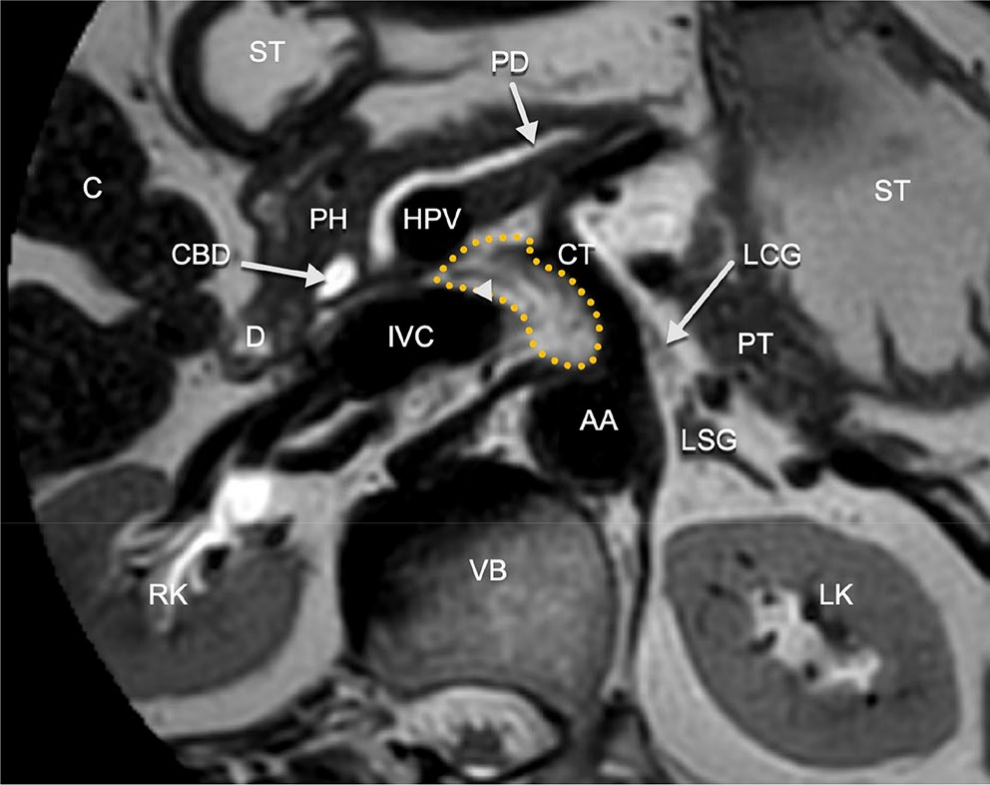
T2-weighted magnetic resonance image of the pancreatic head. The neural plexus contained by the retropancreatic fascia is visible (dotted yellow area in which several linear structures (arrow head) are visible corresponding to nerve fibers of the plexus). The left celiac ganglion (LCG) is visible to the left side of the celiac trunk (CT). AA: abdominal aorta, C: colon, CBD: common bile duct, D: duodenum (descending part), HPV: hepatic portal vein, IVC: inferior vena cava, LK: left kidney, RK: right kidney, LSG: left suprarenal gland, PD: pancreatic duct, PH: pancreatic head, PT: pancreatic tail, ST: stomach, VB: vertebral body
